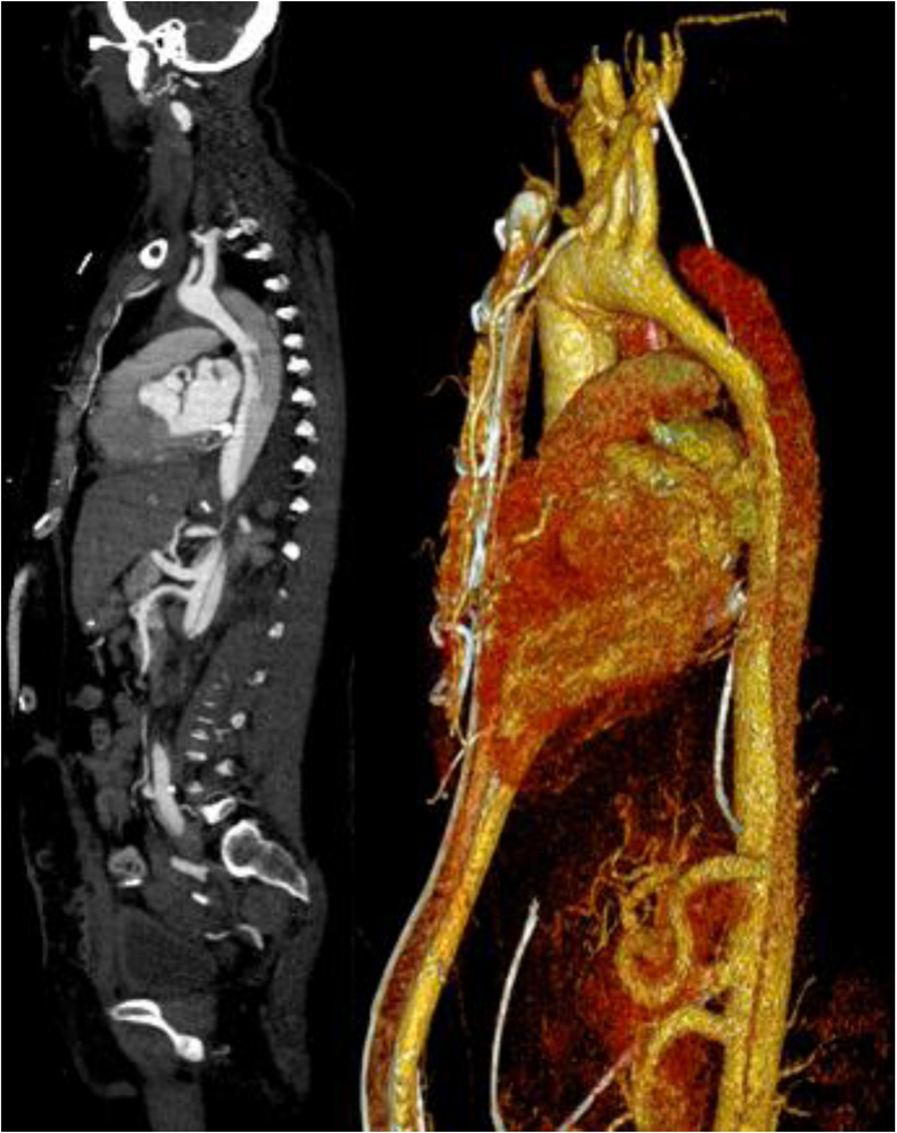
CT scan demonstrating entry site distal to the left subclavian artery
